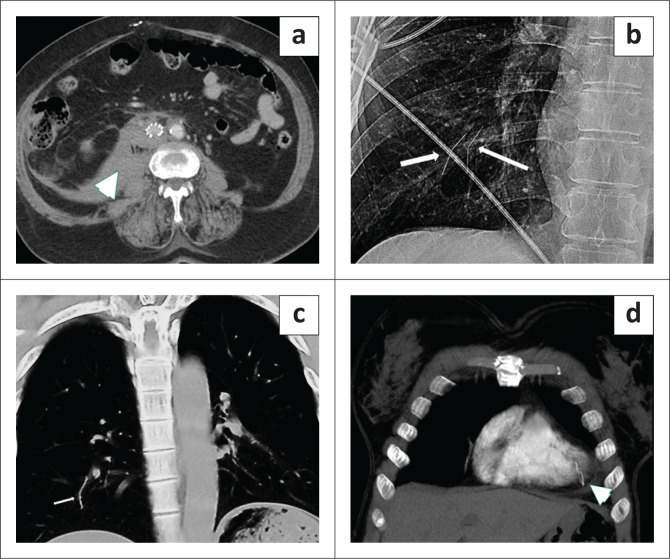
(a) Axial CT abdomen demonstrating a retroperitoneal haematoma (arrowhead) from perforation of the inferior vena cava (IVC) by the IVC filter. (b, c) Chest X-ray and coronal CT thorax showing migrated IVC filter struts (white thin arrows) in the right lower lobe pulmonary artery branches. (d) Embolism of the IVC filter strut into the right ventricle (arrowhead).
